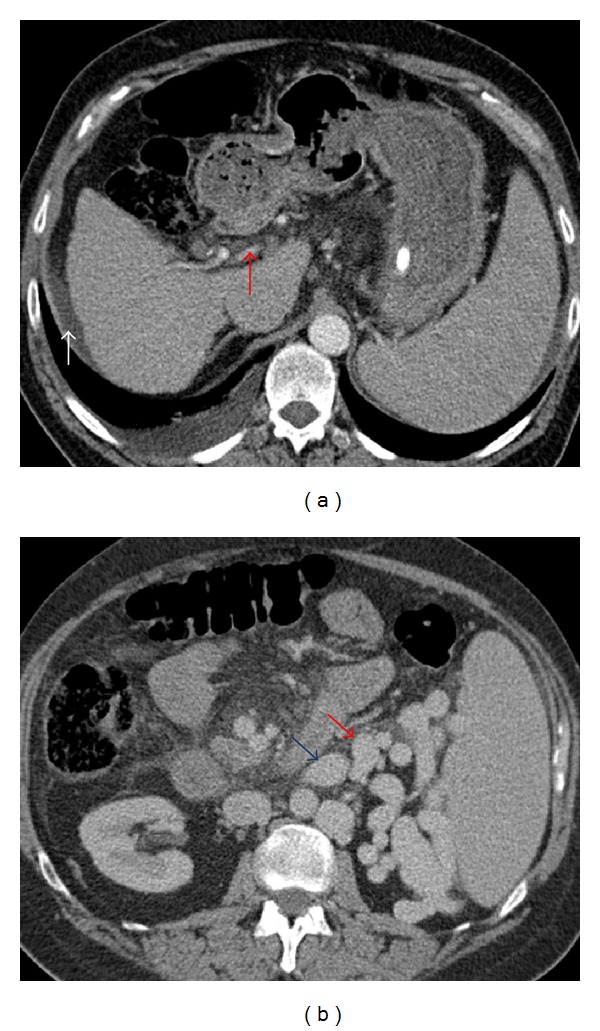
(a) Axial contrast-enhanced CT demonstrates diminutive portal vein (red arrow) and minimal perihepatic ascites (white arrow). (b) Axial CT demonstrates enlarged spleen with multiple perisplenic collateral vessels. A large splenorenal shunt (red arrow) is seen arising from a dilated left renal vein (blue arrow).
